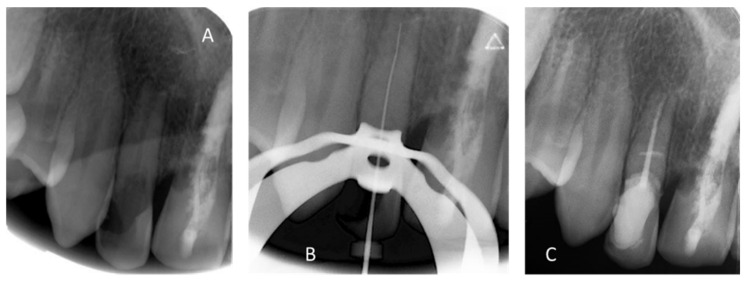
Pre (A), per (B) and post (C) retro-alveolar X-rays of the endodontic treatment of 12 (BioRoot™ and calibrated cone).
